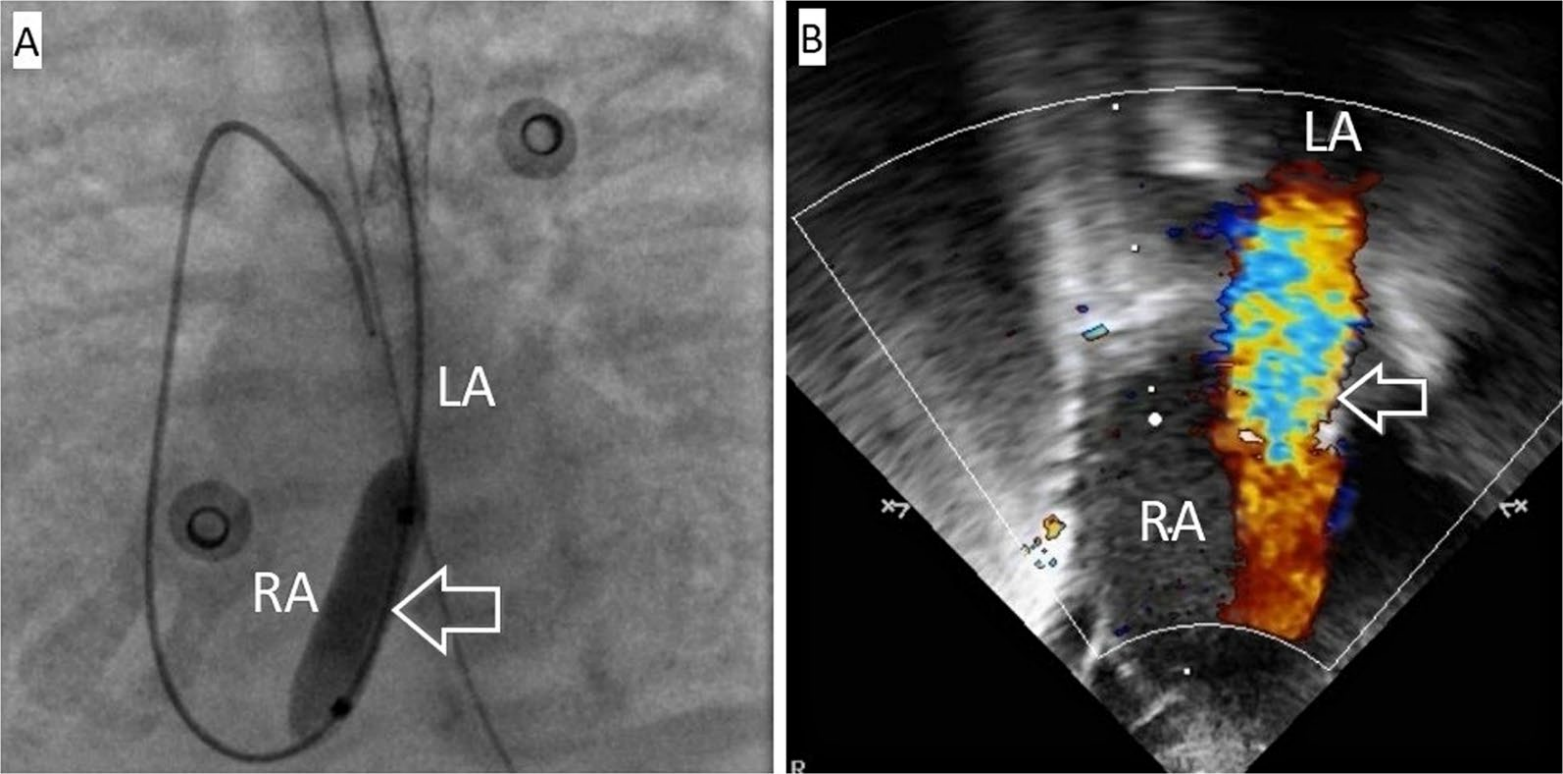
Balloon dilatation of the AS under simultaneous X-ray and Echocardiography. A Balloon septostomy under angiographic control, the balloon (indicated by the arrow) is inflated in the area of ​​the atrial septal defect, anteroposterior view. B Echocardiography after balloon septostomy. Interatrial communication is 3 mm in size (indicated by an arrow). *LA* left atrium, *RA* right atrium
